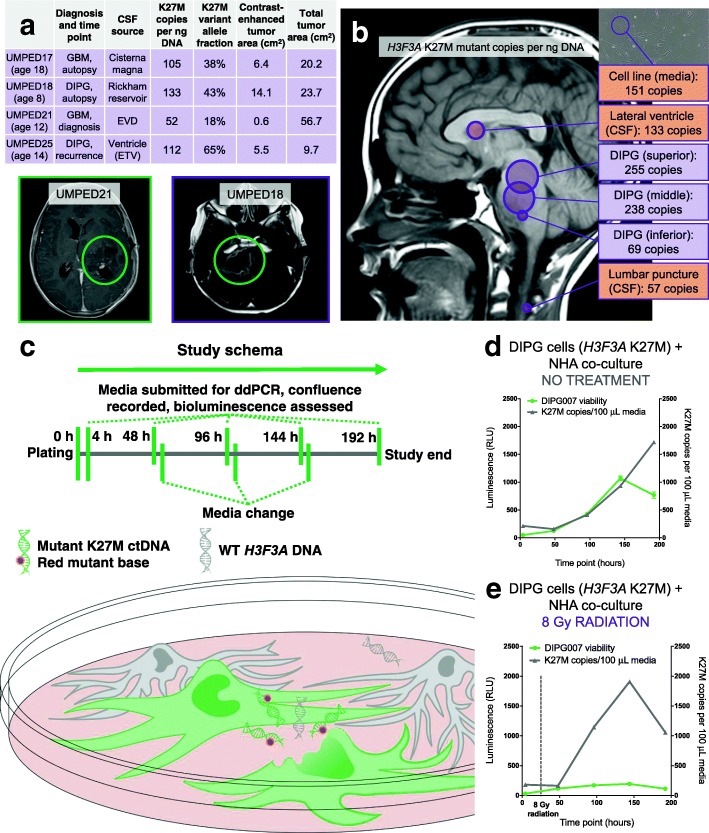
a CSF ddPCR results from experimental samples correlated with contrast-enhancing and total tumor cross-sectional area on MRI. b ddPCR of multi-focal sampling shows K27M copy number varies between tumor (purple) and CSF (orange) regions c Co-culture scheme of bioluminescent human DIPG007 cells with NHAs. d DIPG007 cells release ctDNA in proportion to their proliferation. e 8 Gy radiation results in an increase in mutant ctDNA from DIPG007 cells
